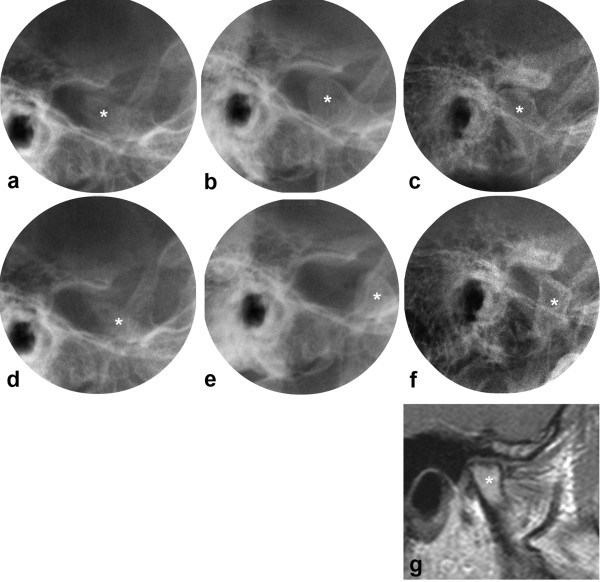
JIA patient with greatly improving disease course in the TMJs, left joint (same patient as in Fig. 5). Transcranial radiographs at closed (a,b,c) and maximally opened mouth (d,e,f), including MRI (g) at closed mouth. Left joint shown from 5 to 33 yrs with extensive bone abnormality, anterior position of the condyle in the fossa at closed mouth and severely impaired condyle translation at baseline (a,d), improved condyle morphology and translation at 2 yrs follow-up (b,e), and further improvement of joint morphology and condyle position at final follow-up (c,f,g). * = mandibular condyle. Mandibular growth rotation shown in Fig. 2c.
